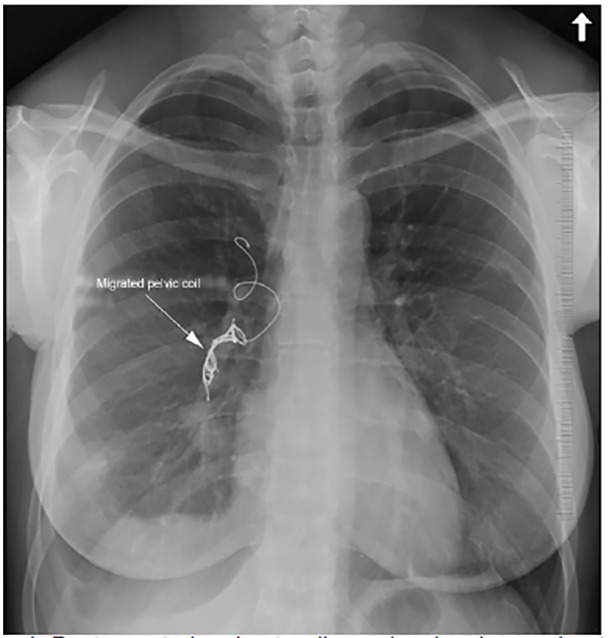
Posteroanterior chest radiography showing a migrated pelvic coil (arrow) in the right pulmonary artery extending into segmental branches.
